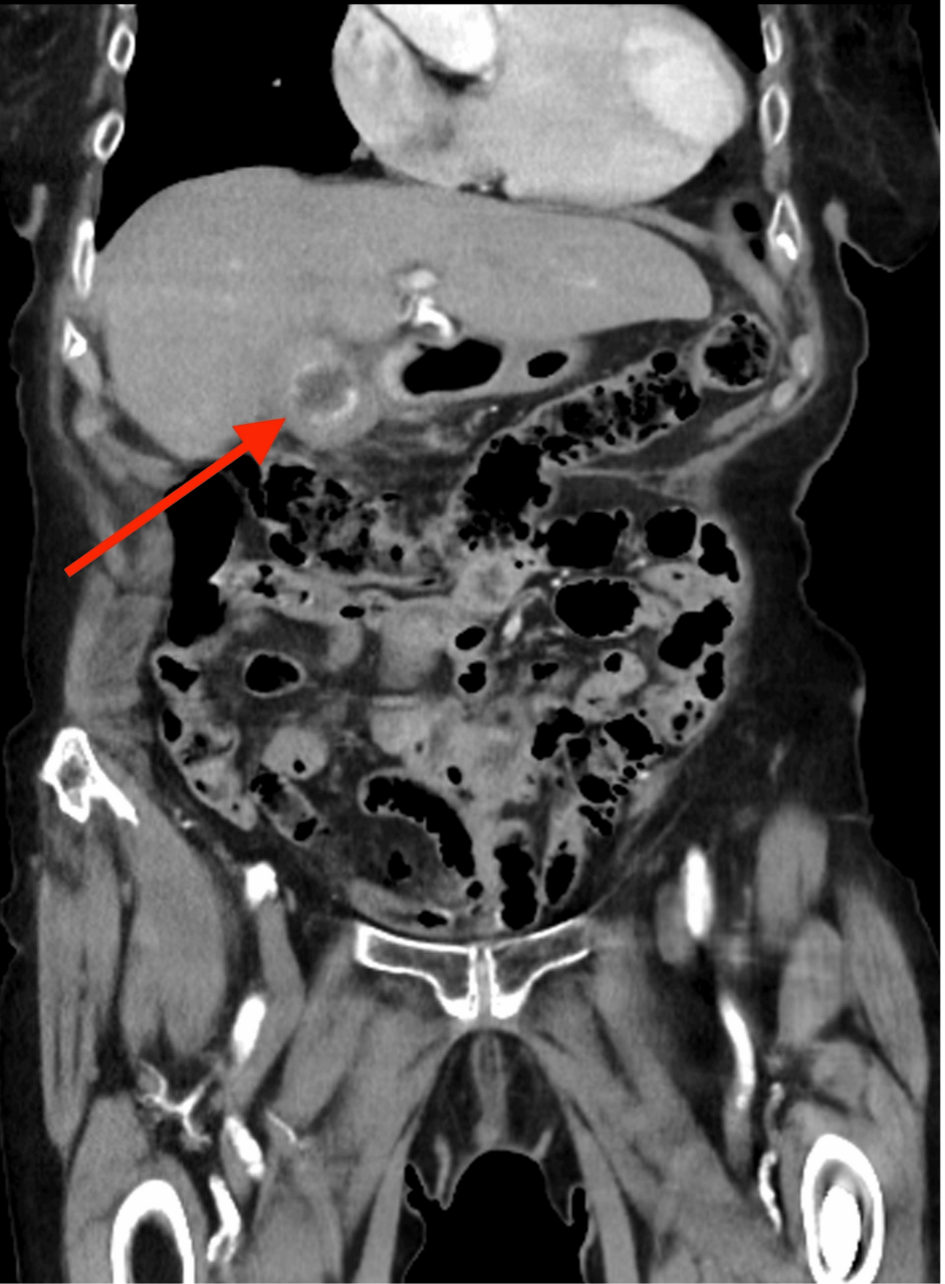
CT abdomen and pelvis (coronal view) with IV contrast demonstrating a contracted thick-walled gallbladder with a retained calculus (red arrow) CT: computed tomography; IV: intravenous
